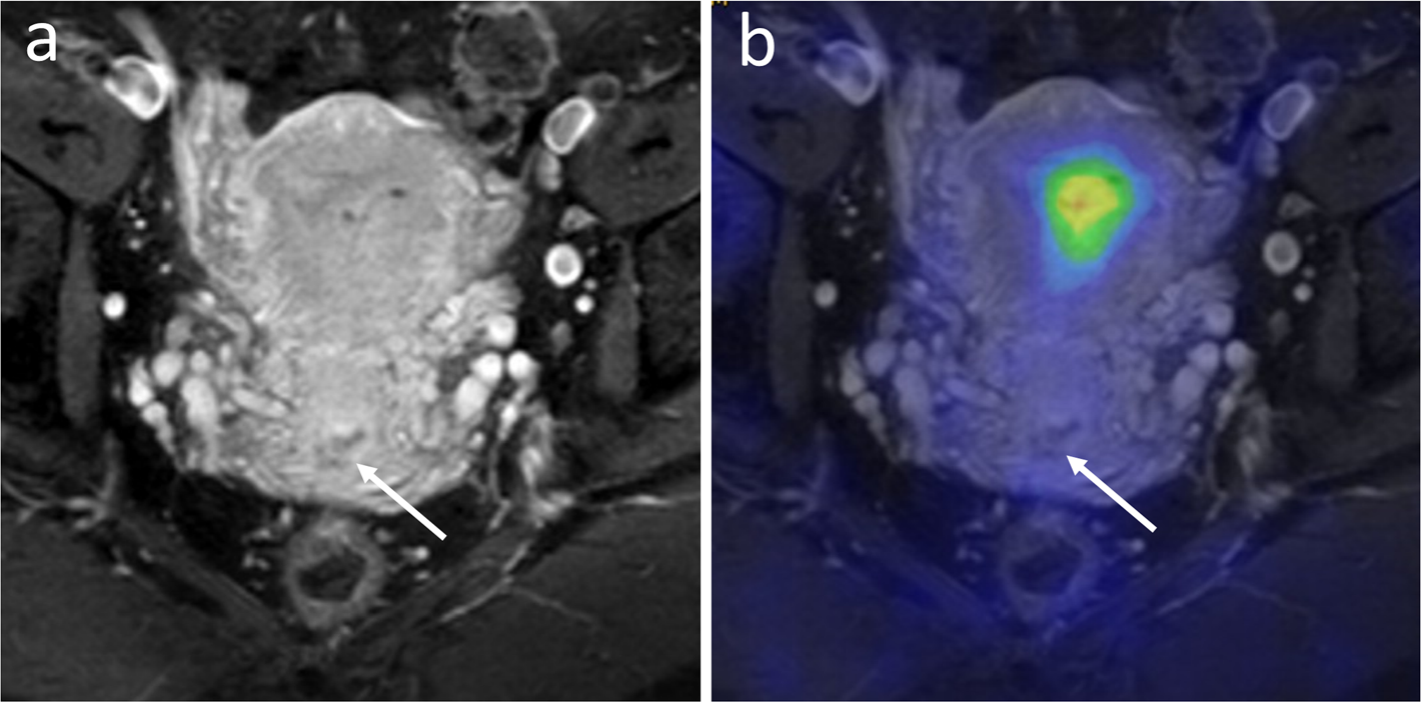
A 38-year old woman with stage T0 cervical cancer at hysterectomy that was misinterpreted as stage T1b1 on MRI. Coronal contrast enhanced T1-weighted image shows a hypoenhancing area in the cervix interpreted as a small residual following loop electrosurgical excision procedure (a, white arrow). PET/MRI shows no FDG uptake confirming post-procedural changes without evidence for a residual (b) (patient no. 9 on Table 2)
